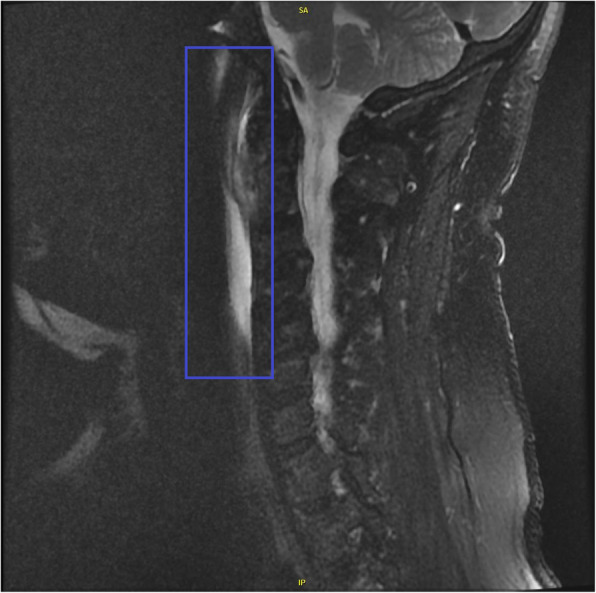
MRI T2 Fat Saturated sequence of cervical spine shows high-signal intensity in the soft tissues representing inflammatory edema of the prevertebral muscles and elongated retropharyngeal effusion (blue rectangle)
